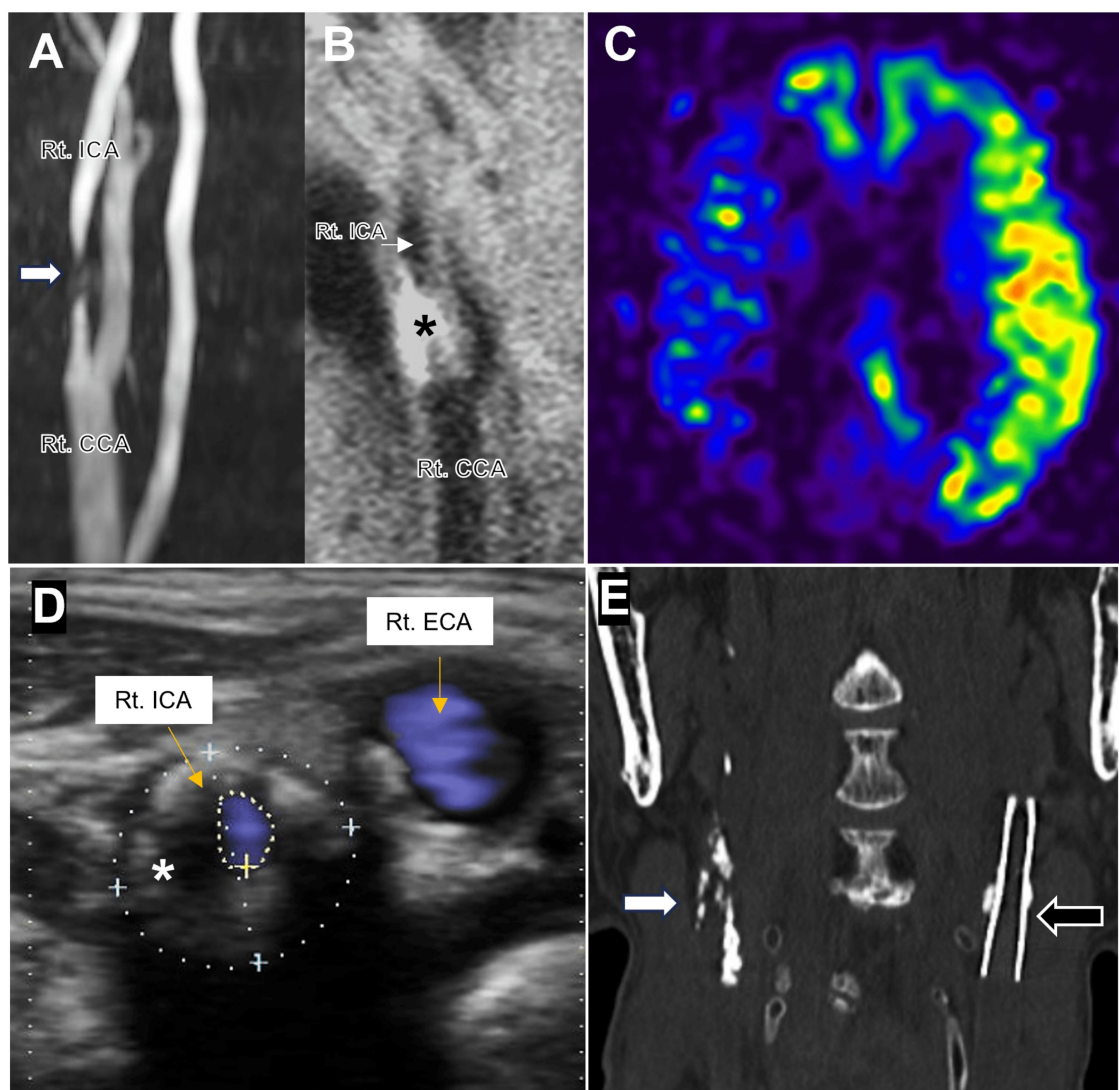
Radiological images at the second intervention (A) Cervical MRA shows severe stenosis with wall irregularity in the right ICA (white arrow). (B) Black-blood T1-weighted image reveals a heterogeneous signal pattern consistent with characteristics of an unstable vulnerable plaque (asterisk). (C) Arterial spin labeling MRI reveals reduced cerebral blood flow in the right hemisphere. (D) Carotid duplex ultrasonography depicts severe luminal stenosis in the right ICA (92% area reduction, peak systolic velocity of 330 cm/second), with a mixed echogenic plaque (asterisk). The blue dotted line outlines the vascular wall, while the yellow dotted line delineates the luminal contour. (E) Coronal slice of the non-contrast cervical CT scan discloses a heavily calcified plaque (white arrow) with scattered small calcifications at the right carotid bifurcation. Stent placement is identified in the left ICA (black arrow). CCA: common carotid artery, ICA: internal carotid artery, ECA: external carotid artery; MRA: magnetic resonance angiography; CT: computed tomography; MRI: magnetic resonance imaging
